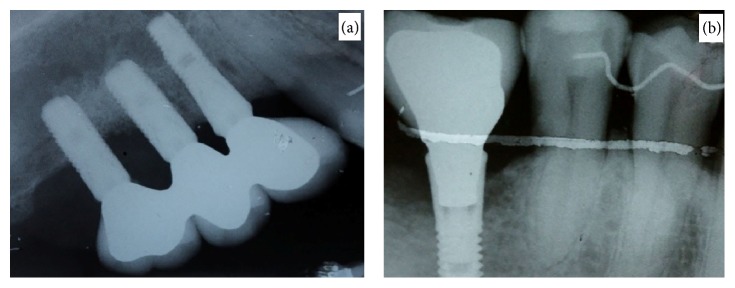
(a) Periapical radiograph that shows bone loss in implants 16 and 17. (b) Periapical radiograph that shows bone involvement in implant 36.
